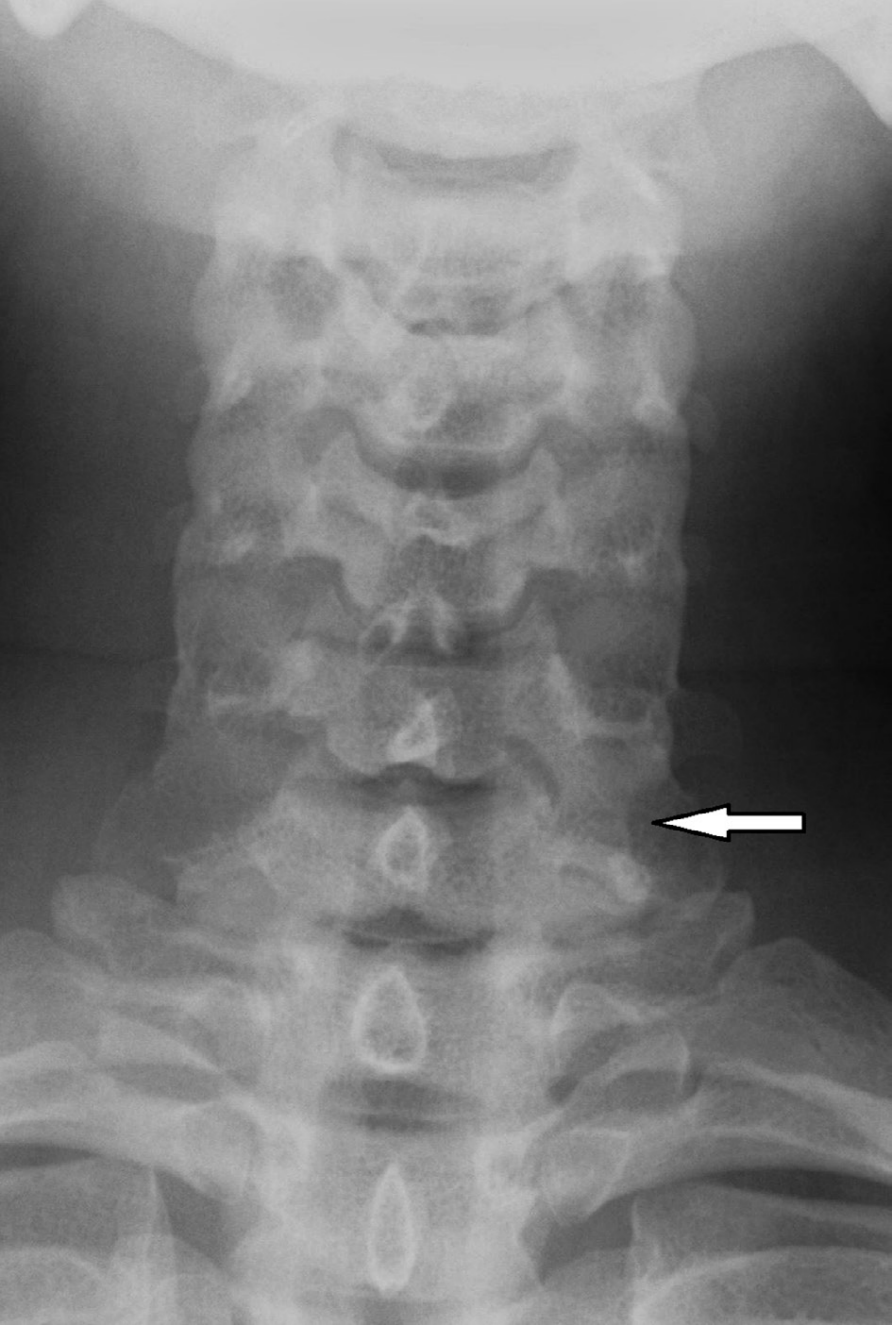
Anteroposterior radiograph of the cervical spine in a 28-year-old female following a motor vehicle accident. It shows a bony projection extending anterior to the C6/C7 vertebral bodies with a radiolucent line between the lateral masses.
